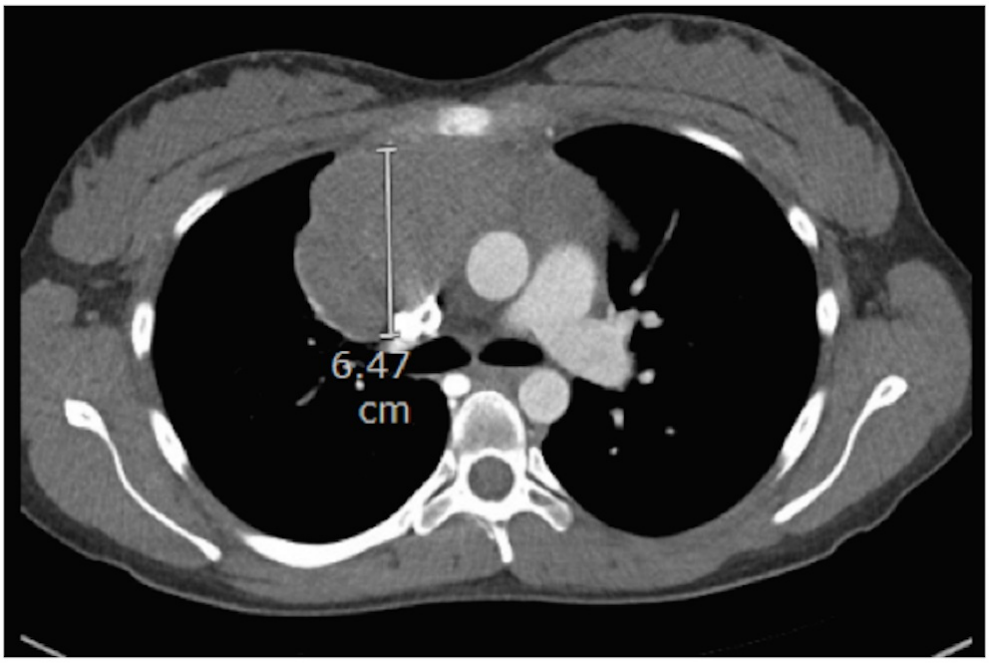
CT chest showing an anterior mediastinal mass measuring 6.47 cm
